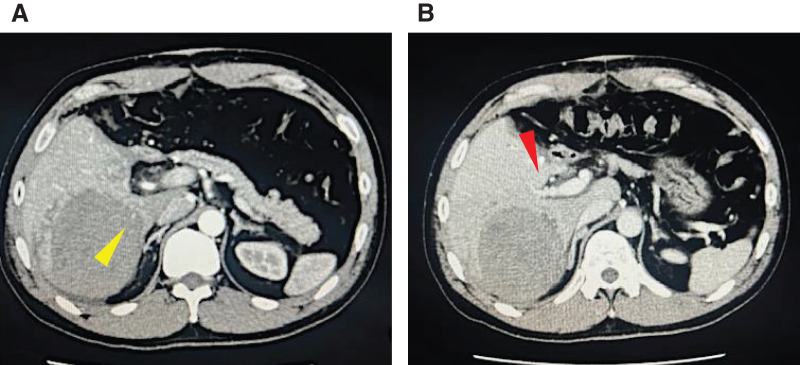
Contrast-enhanced CT scan on POD 3 following extended left hemihepatectomy. (A) In the arterial phase, a large hematoma with contrast medium extravasation (yellow arrow) was observed in the posterior segment of the liver. (B) In the portal venous phase, the anterior branch of the portal vein (red arrow) was compressed and narrowed by the hematoma.
